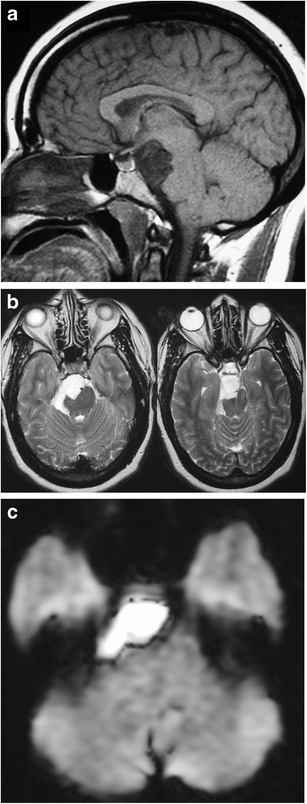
Epidermoid. Sagittal T1WI (a) shows an extra-axial lesion along the endocranial surface which has heterogeneous mostly low-intermdiate signal, and on T2WI has high signal (b). Diffusion-weighted imaging (DWI) (c) shows restricted diffusion
